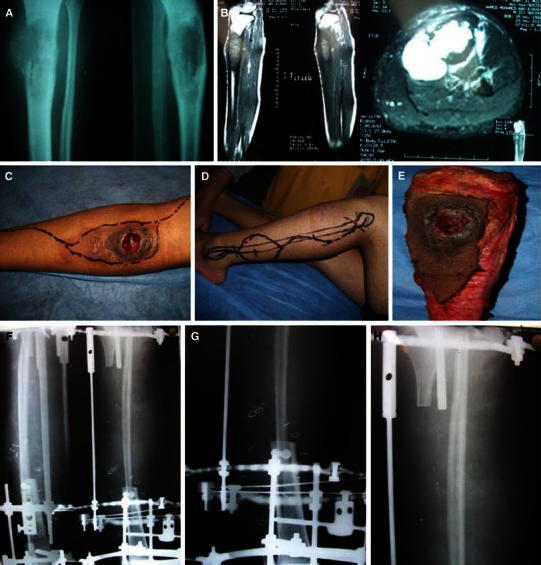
Male patient 22 years old with bone sarcoma of the tibia. a Preoperative plain X-ray; b preoperative MRI for diagnosis and staging; c, d intraoperative photos showing skin incision planning for tumor resection and fibula harvest; e intraoperative photo of tumor mass after resection; f plain postoperative X-ray with Ilizarov fixator applied; g, h plain X-ray taken three months postoperatively
